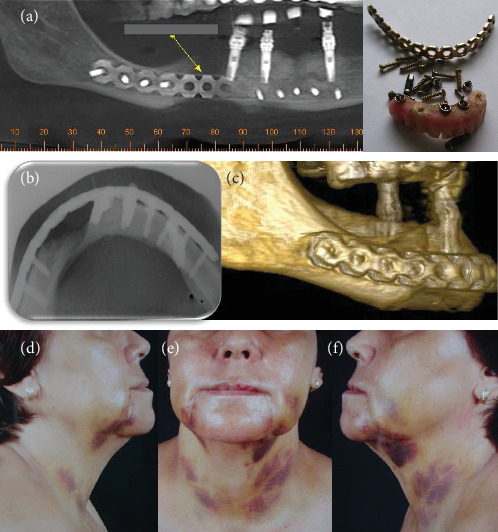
(a) CBCT scan shows the reconstructive plate associated with the radiolucent destruction area in the right quadrant of the mandible. (b) Reconstructive plate, implants, screws, and prosthesis removed from the mandible with infection. (c) Occlusal radiograph with the large radiolucent area. (d) 3D reconstruction of the right quadrant of the mandible. (e, f) Clinical appearance after hospital discharge.
